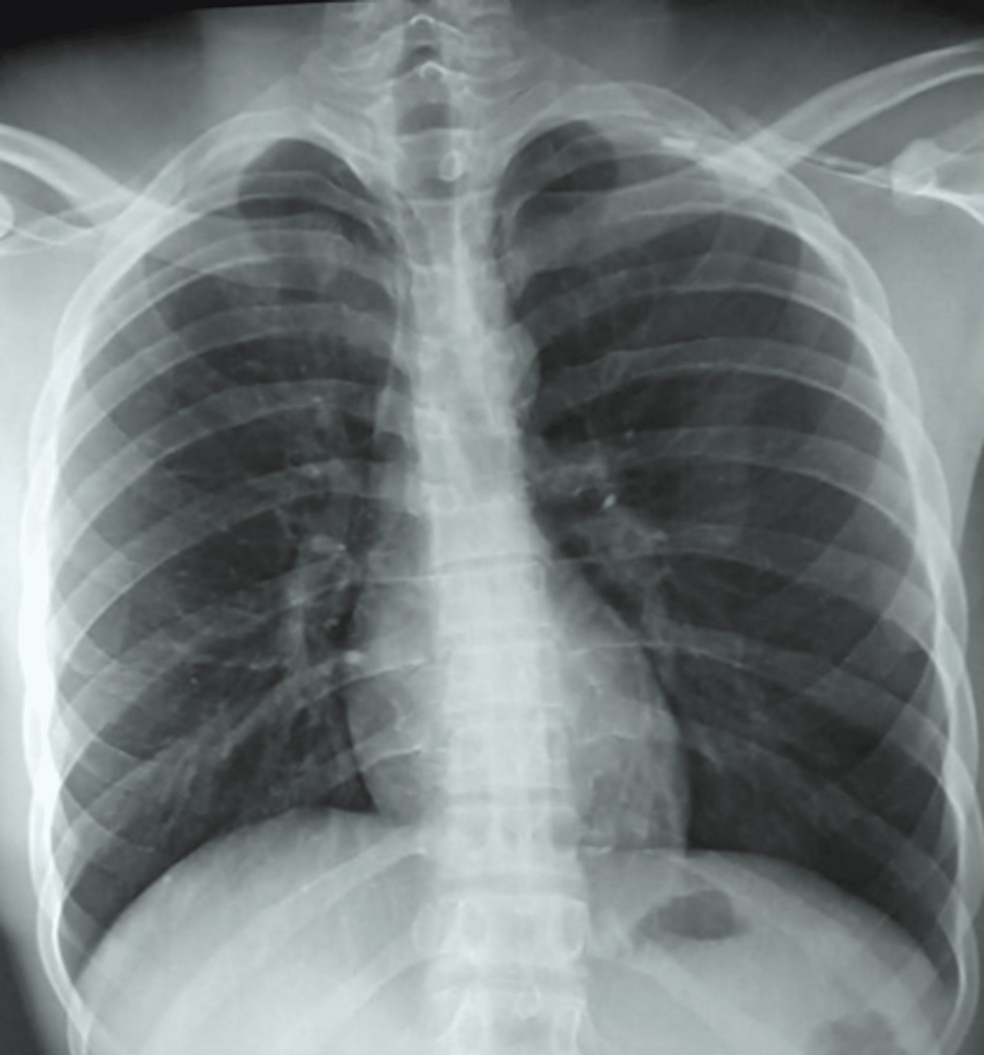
X-ray Thorax The image shows normal thoracic radiography outside of a thoracic distension.
